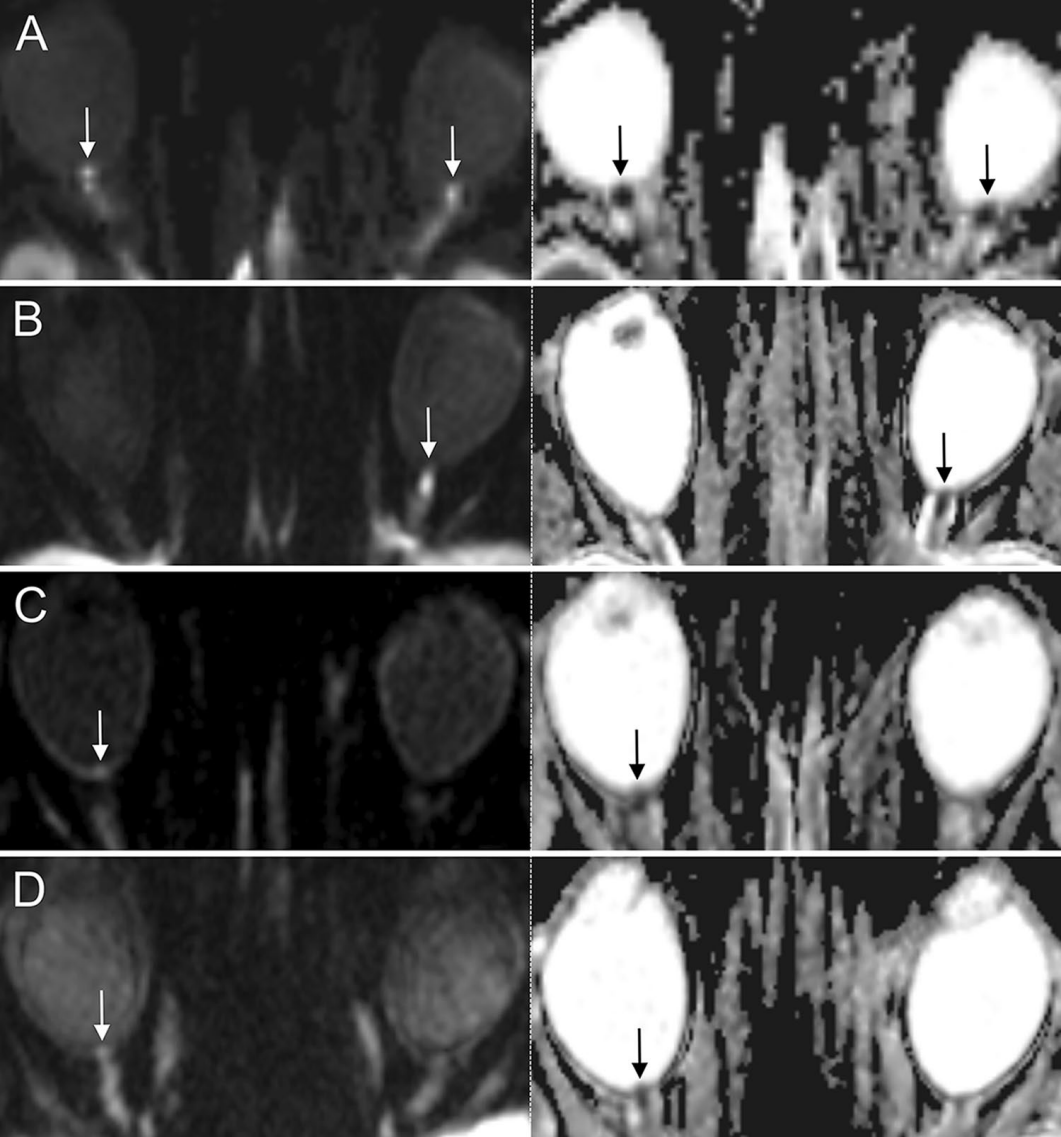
Examples of 3 T DWI-MRI in 4 GCA patients presenting with acute arteritic AION (left row: DWI b = 1000 s/mm2, right row: corresponding ADC images). (A, B): Distinct findings of ONH restricted diffusion in 2 patients with AION (white arrows; bilateral (A) and left-sided (B)). Note the marked qualitative ADC reduction in both cases (black arrows). (C, D) Subtle findings of ONH restricted diffusion (white arrows) and corresponding visually qualitative ADC reduction (black arrows) in 2 patients with right-sided AION. Abbreviations: AION, anterior ischemic optic neuropathy; DWI-MRI, diffusion-weighted magnetic resonance imaging; GCA, giant cell arteritis; ONH, optic nerve head.
